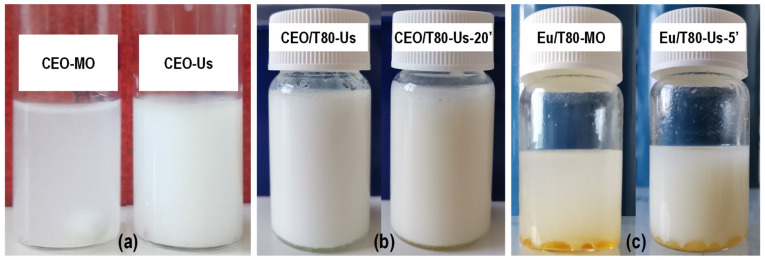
The visual aspect of (a) clove essential oil (CEO) dispersions in acetic acid solutions obtained without emulsifier by mechanical homogenization (CEO-MO) or ultrasonication (CEO-US); (b) CEO dispersions with emulsifier Tween 80 immediately (CEO/T80-Us) and after 20 min (CEO/T80-Us-20’) from ultrasonication; and (c) eugenol (Eu) dispersions in acetic acid solution obtained with emulsifier by mechanical homogenization (Eu/T80-MO) or ultrasonication (Eu/T80-Us-5’).
